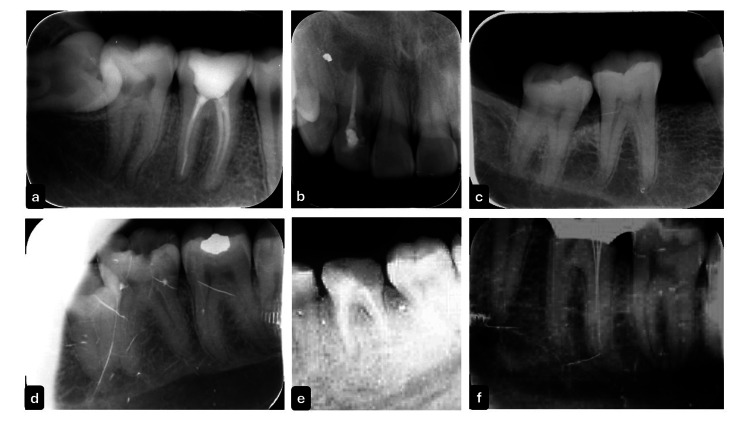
Diagnostically Acceptable and Unacceptable IOPA Radiographs. a-c) Diagnostically acceptable images demonstrating proper contrast, positioning, angulation and processing, d-f) Diagnostically unacceptable images due to issues such as poor positioning, incorrect contrast and improper angulation. IOPA: intraoral periapical
